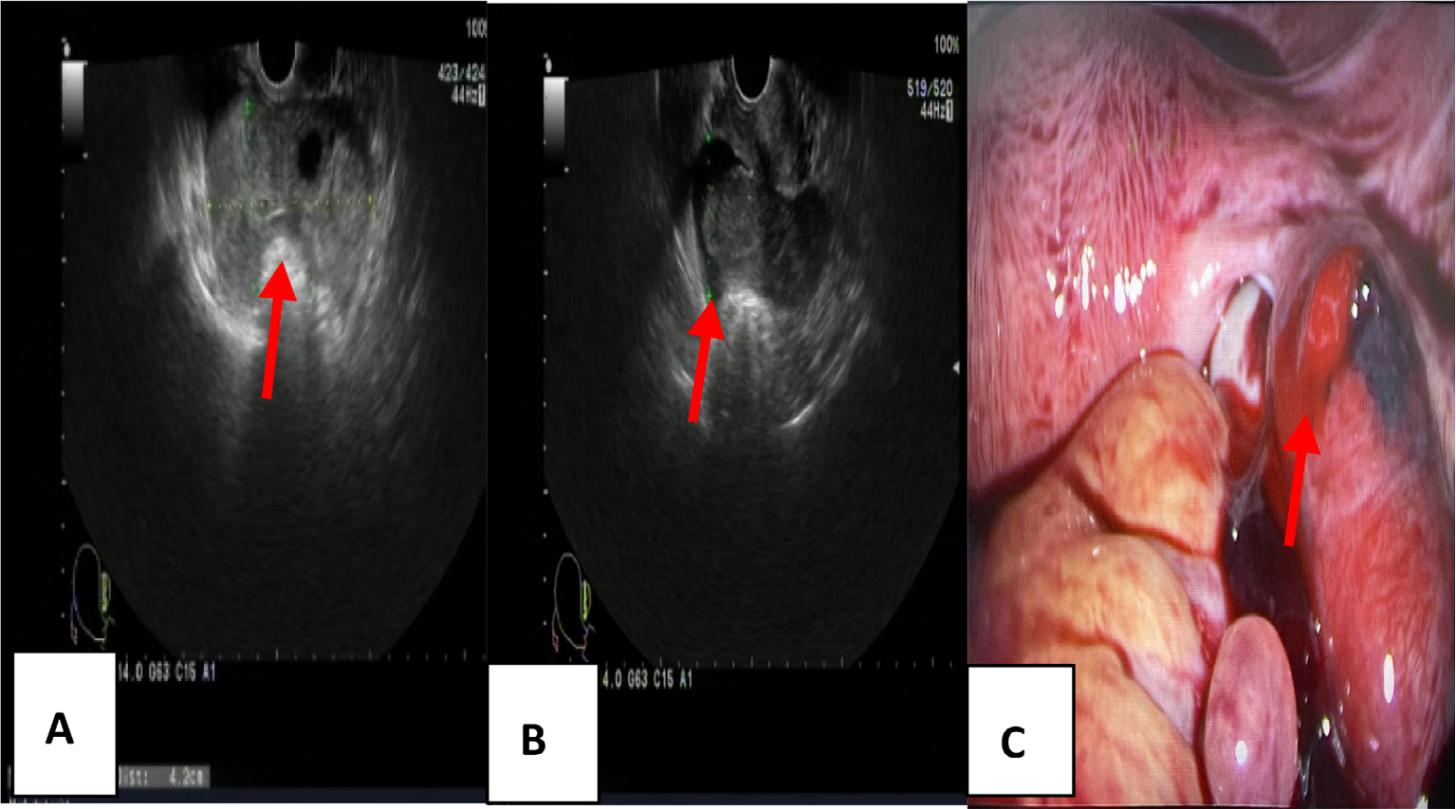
A 30‐year‐old women with RTP. A, Transverse sonogram showing a more mixed mass in the left fallopian tube; B, pelvic fluid volume; C, intraoperative photo displaying a visible rupture on the surface of the left fallopian tube.
